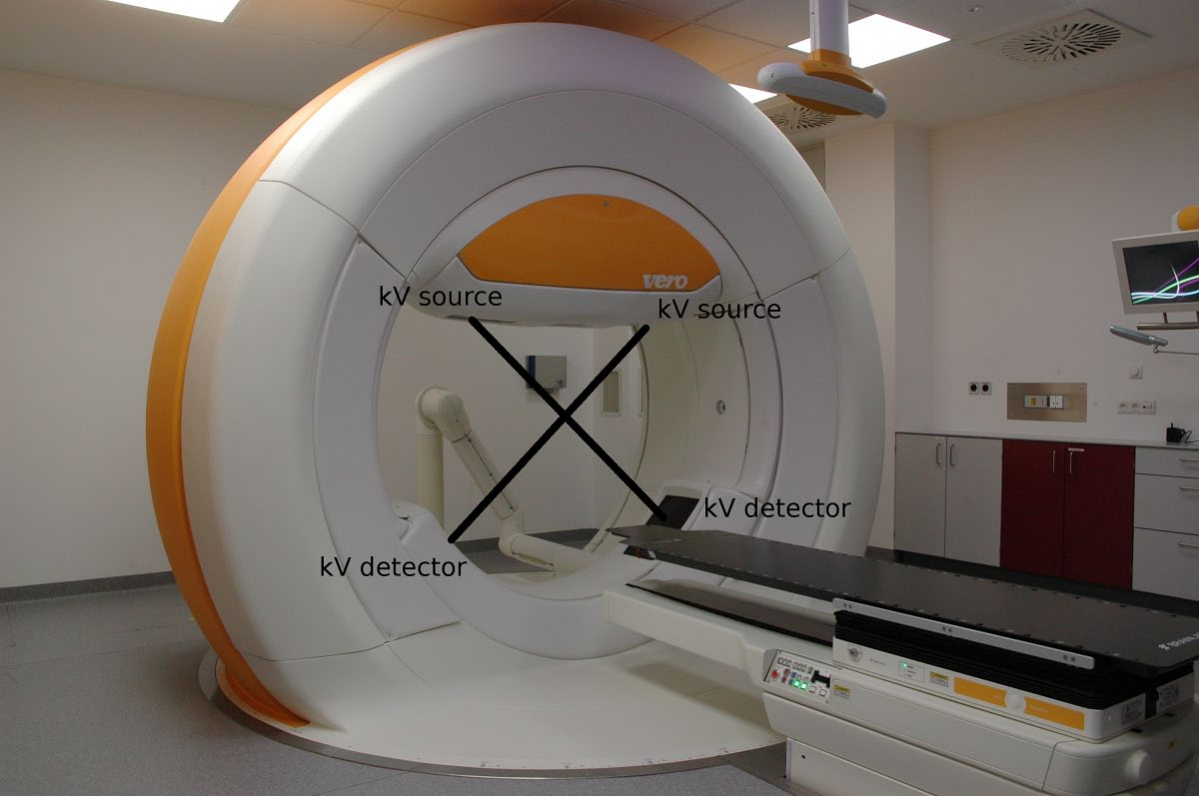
A Vero system equipped with two kV imagers for CBCT.
